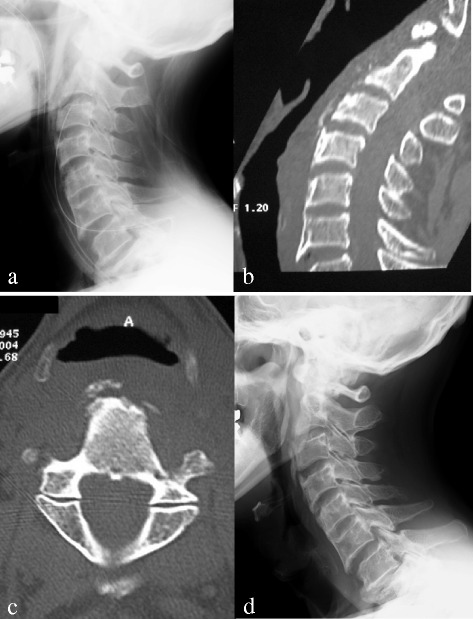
a Lateral radiograph after the second resection of the ossification of the anterior longitudinal ligament also demonstrated decreased compression of the esophagus. b, c Sagittal and axial computed tomographic images of the cervical spine (C3, bone window) after the second resection of the ossification of the anterior longitudinal ligament showed significantly decreased ossification and osteophytes without compression of the esophagus. d There was no recurrent ossification of the anterior longitudinal ligament with anterior osteophytes compressing the esophagus 1.5 years after the second resection on a lateral radiograph
